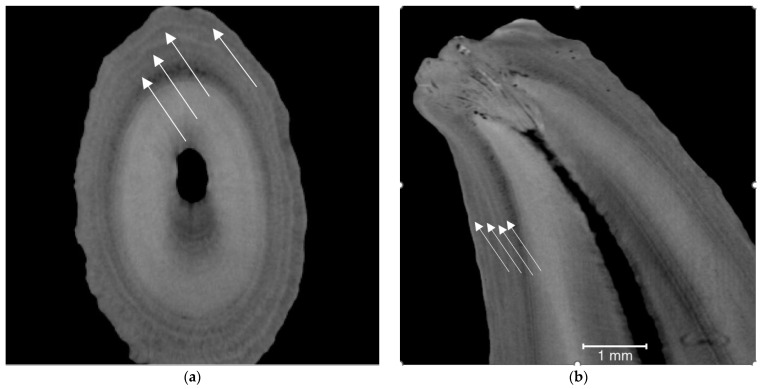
Two-dimensional images of fox tooth stored at room temperature for only 3 years before µ-CT: (a) transverse; (b) longitudinal. The fox was estimated to be 4 years old. Arrows point to dark layers.
